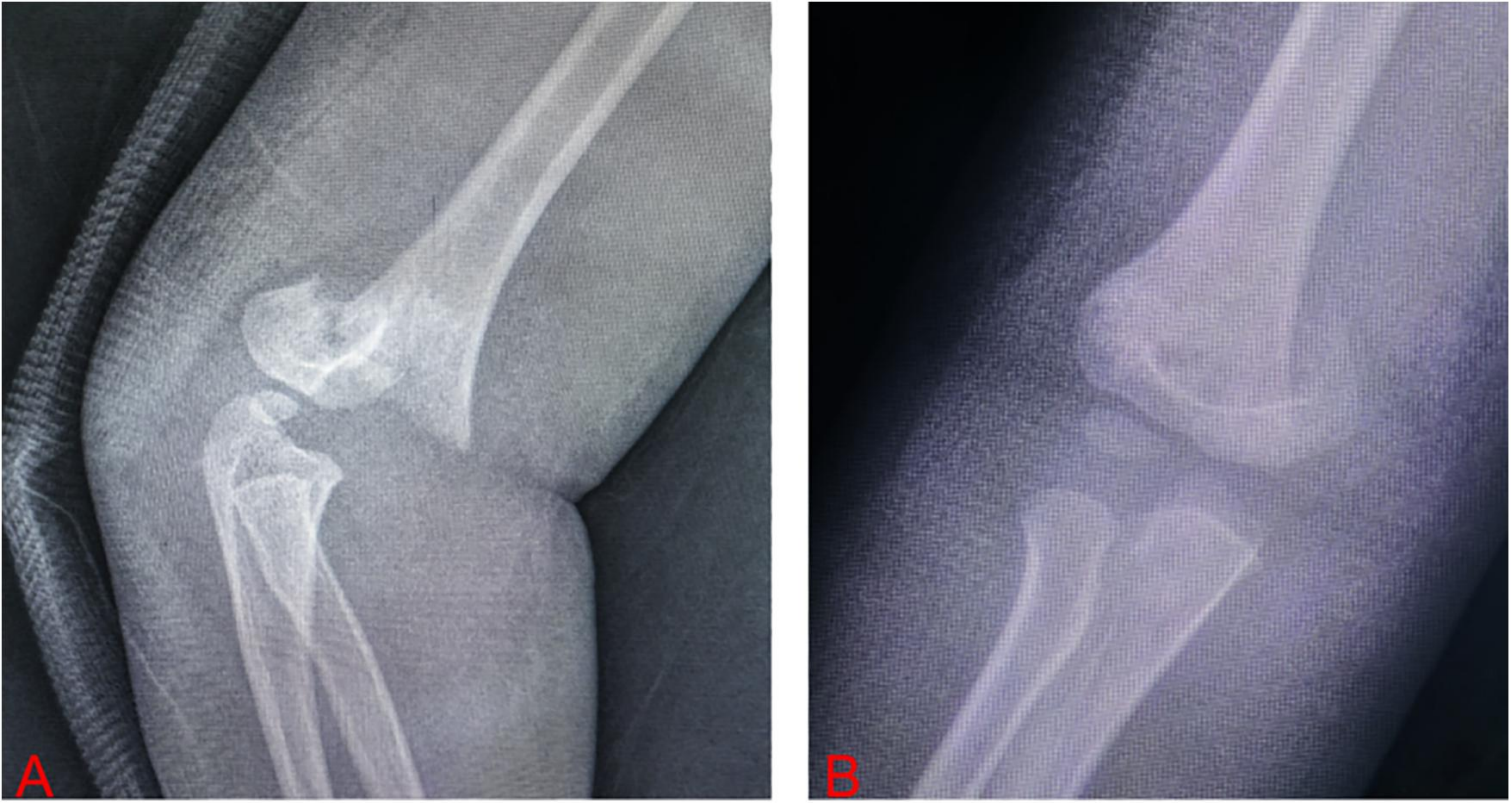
A 4-year-old female patient with a Gartland type III supracondylar humeral fracture. (A) Shows lateral x-ray of the elbow joint, and (B) shows the anteroposterior x-ray of the elbow joint.
